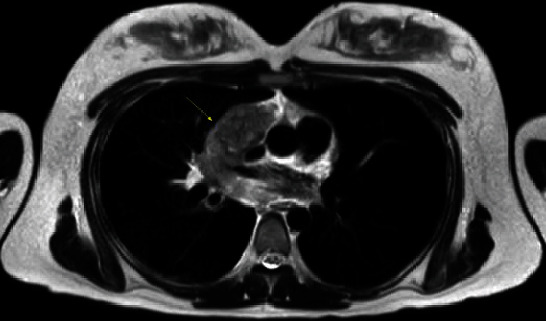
T2-weighted sequence: anterior mediastinal mass consisting of matted lymphadenopathy.
